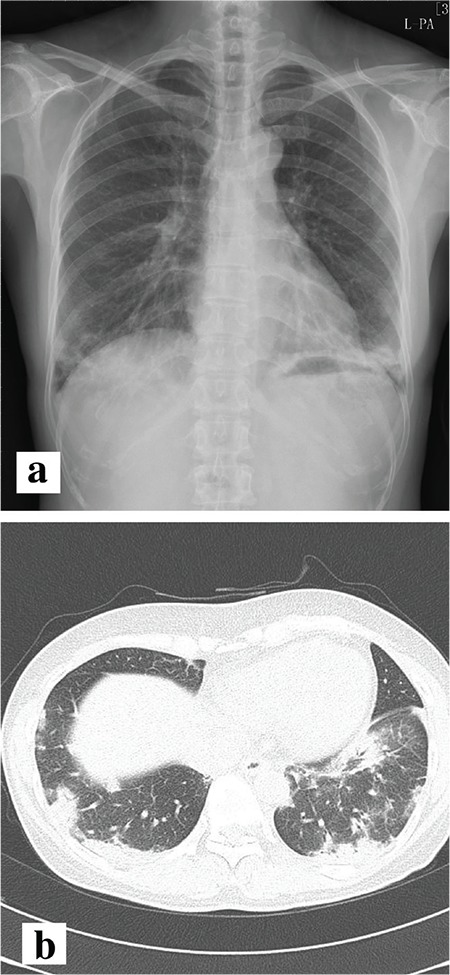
The initial chest X-ray showed pneumonic consolidations in both lower lung fields (a). Chest computed tomography showed patchy subpleural ground-glass opacities and pneumonic consolidations in the lower lobes of both lungs (b).
